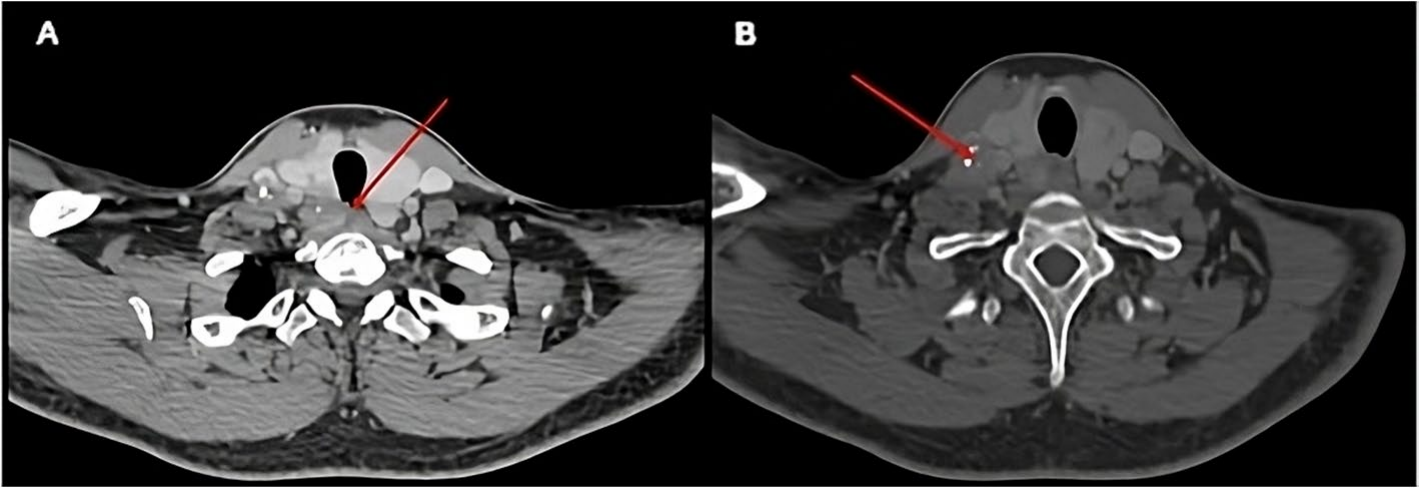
Axial section of CT neck/thorax; red arrow highlights invasion of mediastinal mass into posterior trachea (A). Level IV lymph node compressing the right internal jugular vein (B)
